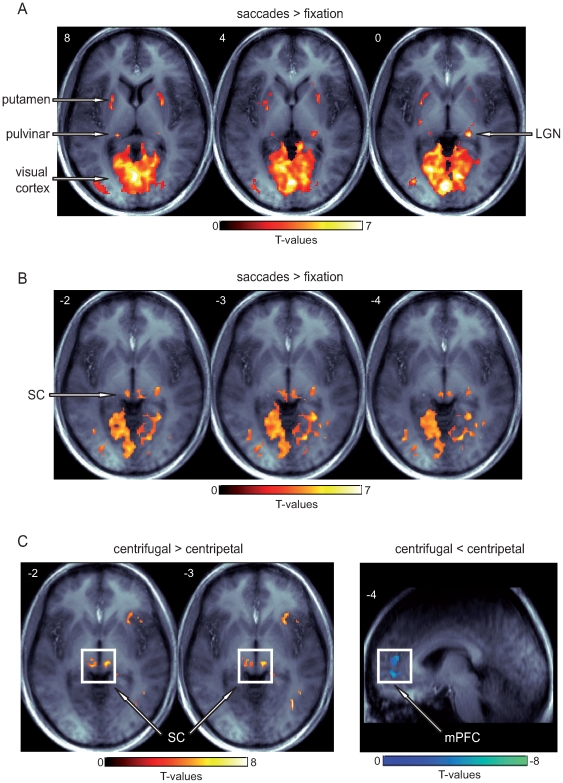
Saccade-related BOLD activity. (A) Saccade execution was associated with robust activity in bilateral medial occipital cortex, putamen, pulvinar, and LGN as compared to cued fixation trials based on the standard HRF model (*6sec-model*). (B) The same contrast derived from the alternative *4.5sec-model* (i.e., optimized for the SC) revealed robust saccade-related activity within both the left and right SC. (C) The direct comparison between centrifugal and centripetal saccades revealed higher activity within the SC (*4.5sec-model*) as well as a stronger prefrontal deactivation for the former (*6sec-model*). Activations are displayed on the averaged T1-weighted image. Display cut off: T>2.5, extent threshold k>15.
